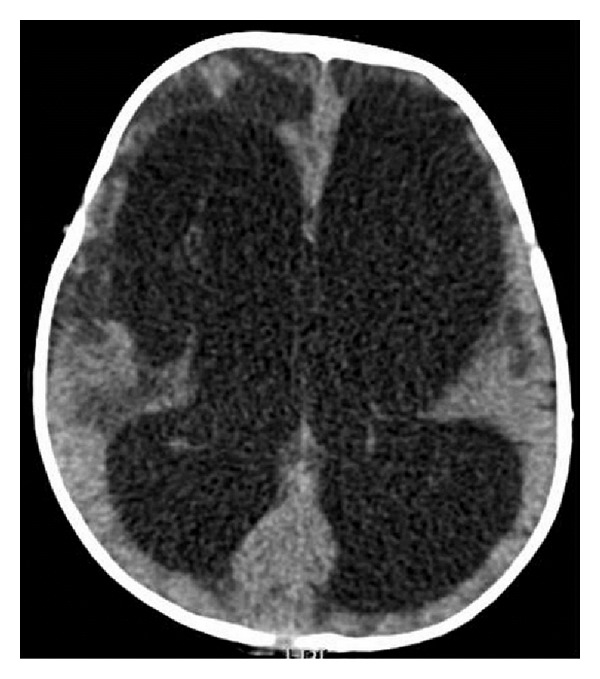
Nonenhanced brain computed tomography scan eight weeks after admission. Axial image shows significant nonobstructive hydrocephalus resulting in compression of cerebral parenchyma. There are hypodensities involving the periventricular regions and the frontal lobes bilaterally with associated cystic encephalomalacia, more on the right.
